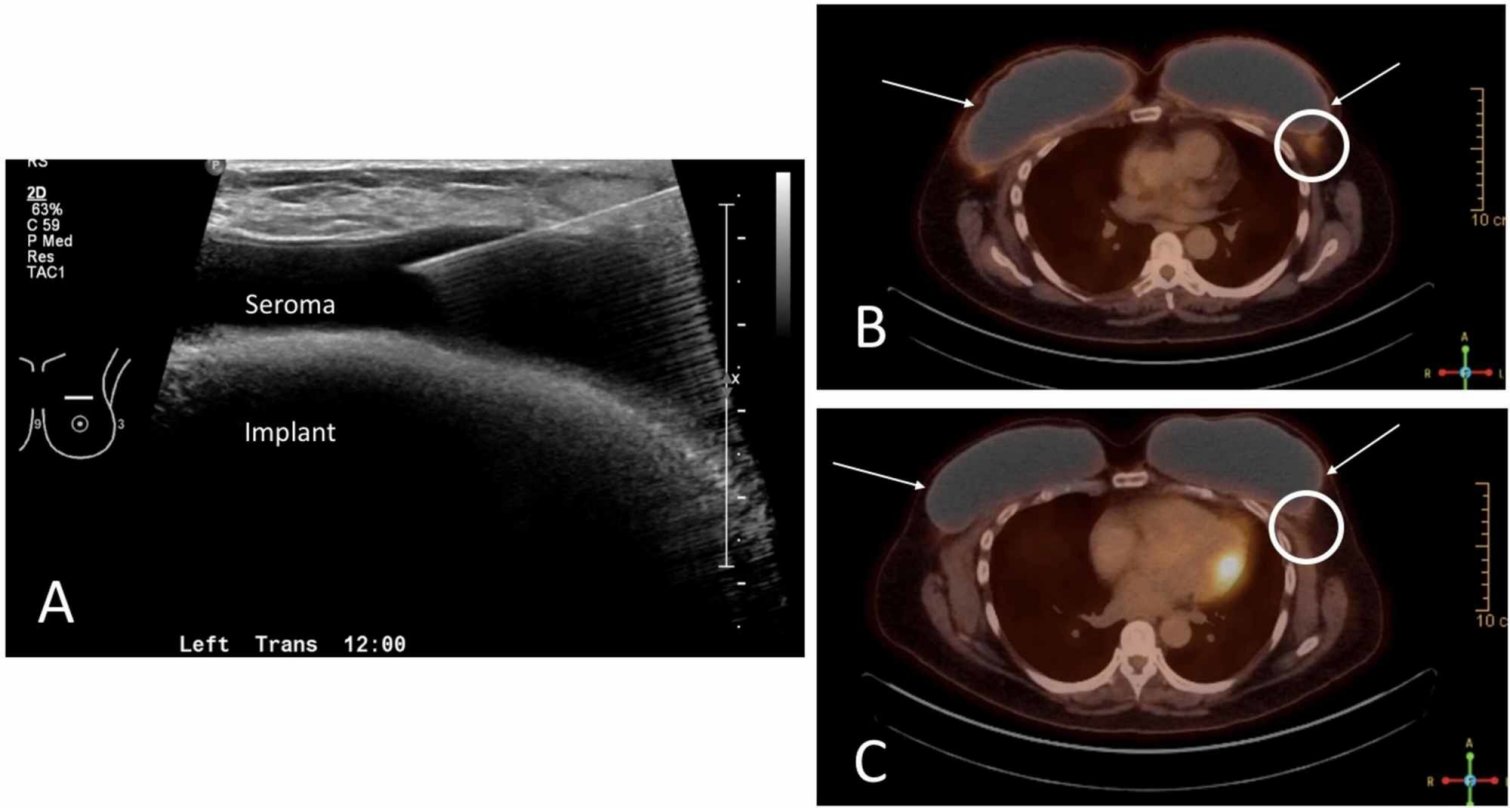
Perioperative imaging (A) Ultrasound-guided aspiration periprosthetic seroma for diagnosis of BIA-ALCL. (B) Immediate postoperative PET scan demonstrating postoperative increased uptake at the surgical site (white arrows) and reactive lymph nodes in the left axilla (white circle). (C) One-year interval PET scan demonstrating reduced surgical site uptake (white arrows) and improved reactive lymph nodes in the left axilla (white circle). BIA-ALCL, breast implant-associated anaplastic large cell lymphoma; PET, positron emission tomography.
